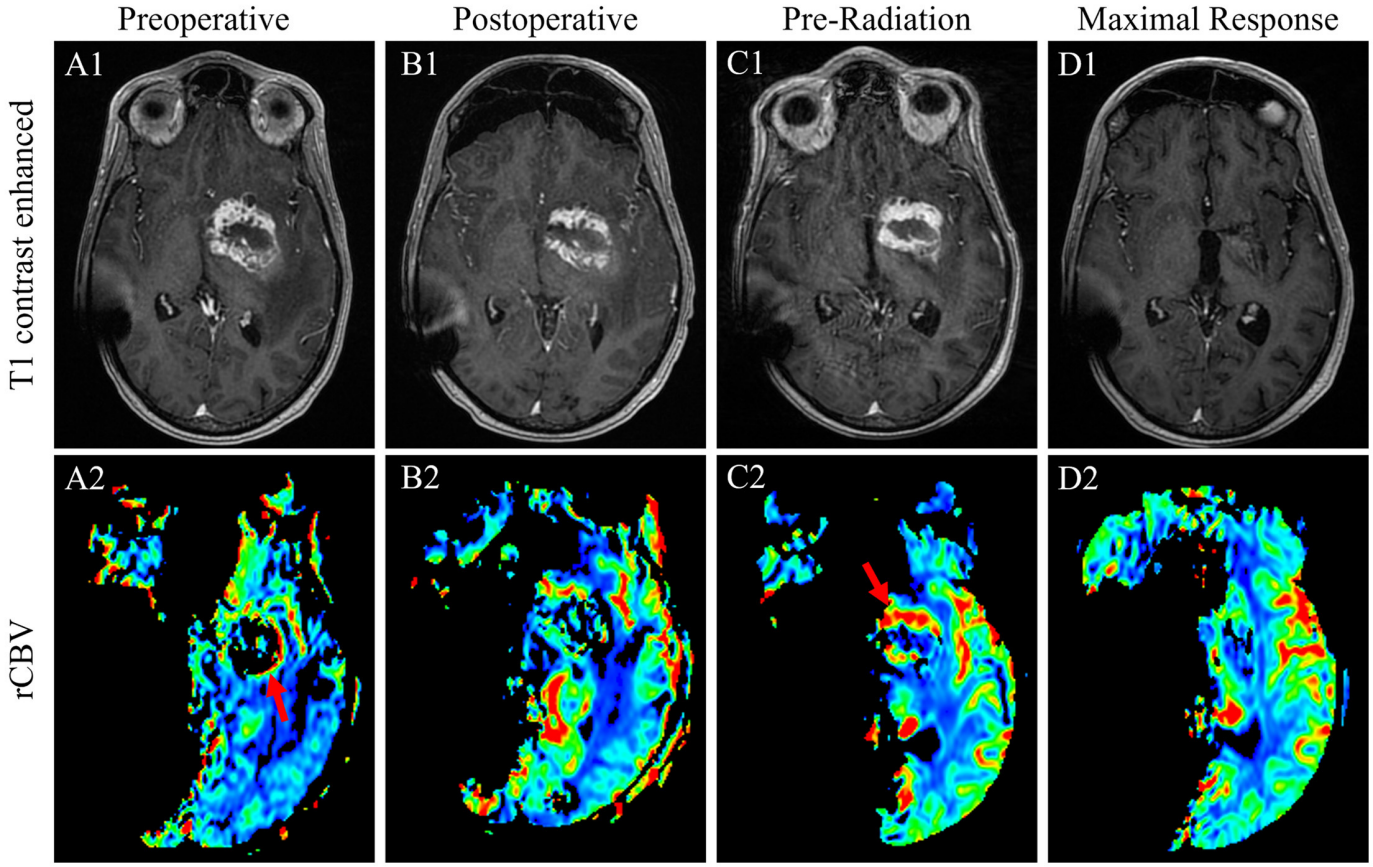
T1-weighted contrasted sequences (1) and relative cerebral blood volume MRI sequences of case 2 patient's GB at the time of initial diagnosis (A); immediate postoperative results following a subtotal resection (B); 1-month postoperative results prior to the initiation of radiation with evidence of substantial persistent disease with the evidence of interval progression (C); and the time of maximal response in the brain with complete disease remission 7 months after the conclusion of radiation therapy (D).
